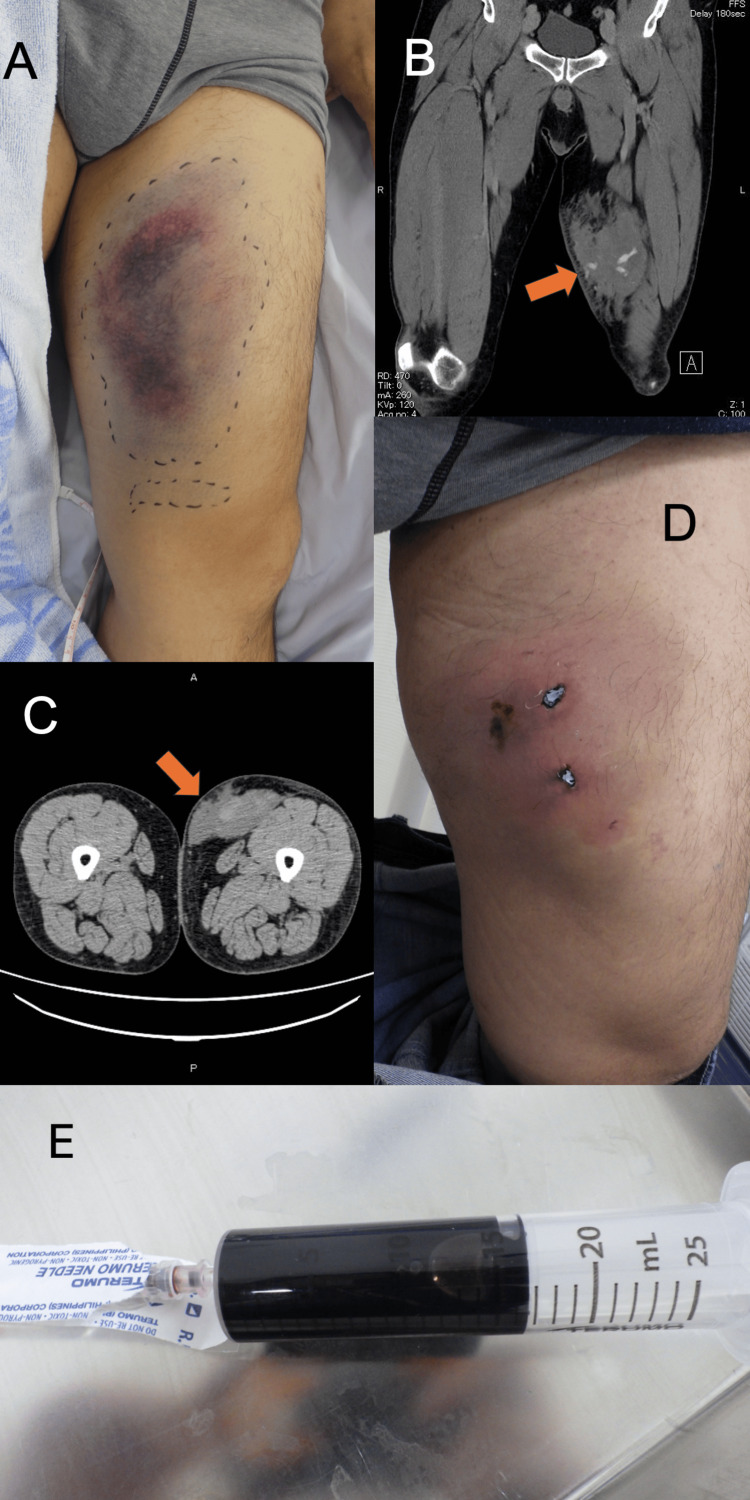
Left thigh Bruising and swelling are seen around the left thigh on the day of admission (A). Coronal contrast-enhanced CT shows a hematoma with extravasation of a branch of the left superficial femoral artery without apparent aneurysms or femoral fractures (B). Axial CT of the left thigh shows a subcutaneous hematoma in the left thigh (C). Orange arrows show the respective lesions. Bruising and swelling with two necrotic plaques are seen around the left thigh 10 days after discharge (D). Hematomatous fluid was drained by needle aspiration (E). Usually, serosanguineous fluid is drained by needle aspiration, but in this case, drainage was performed soon after injury; thus, hematomatous fluid was drained. CT: computed tomography
